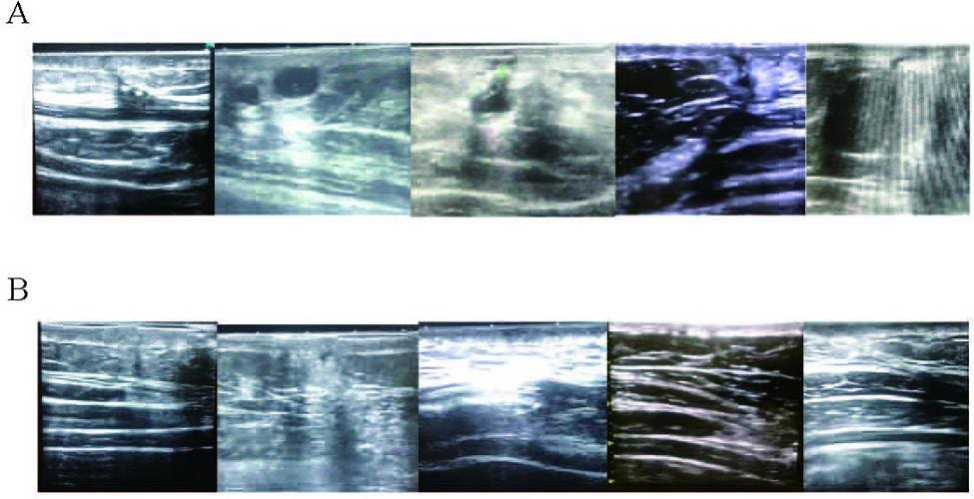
Ultrasound of scars in the control group (A) and the double W group (B)
